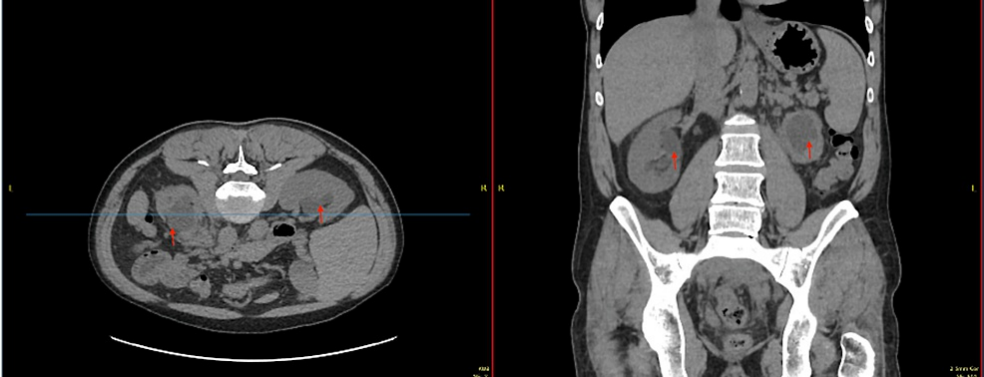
Unenhanced computed tomography scan of kidneys, ureters, and bladder Axial and coronal sections show bilateral hydronephrosis (indicated with red arrows), worse on the left.
